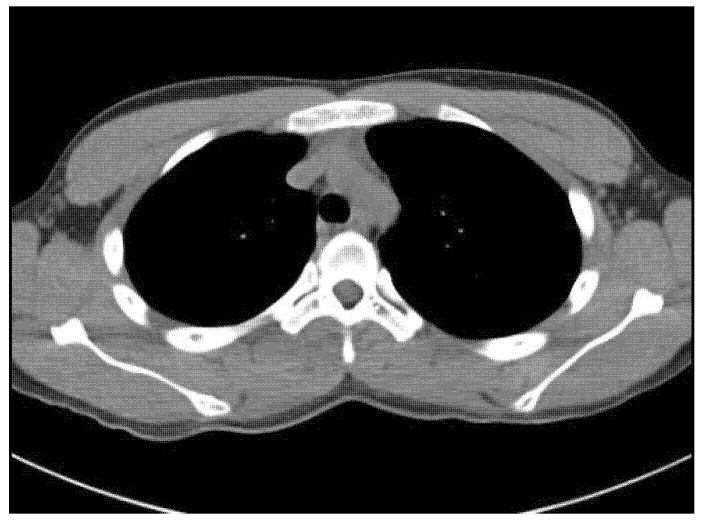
Chest CT taken at an outside hospital about 5 months prior to presentation demonstrates no abnormal mass lesion at the paraspinal area of the thoracic spine.
